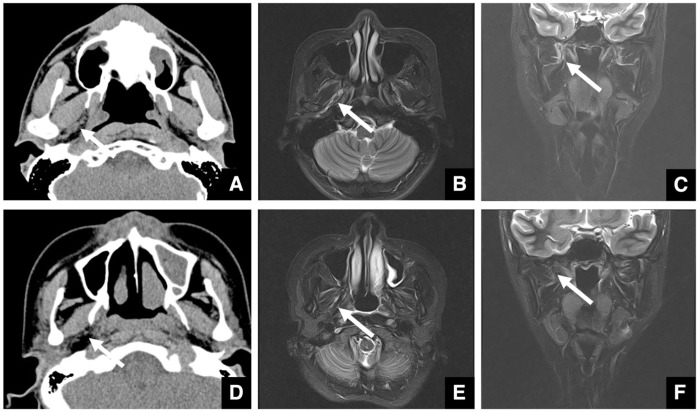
MDCT (slice thickness, 3.0 mm) and MR images of the pterygoid venous plexus. (A) Axial soft tissue MDCT image of a well-developed pterygoid venous plexus. Vascular structures (arrow) are prominent within fat tissue. Mucosal cysts are seen incidentally in the left maxillary sinus. (B) Axial MRI short tau inversion recovery (STIR) image (TR 4700 ms; TE 75 ms; slice thickness 6 mm) of the same patient as in (A). High signal intensity is observed within the fat tissue (arrow). (C) Coronal MRI STIR image of the same patient as in (A). High signal intensity is observed within the fat tissue (arrow). (D) Axial soft tissue MDCT image of an underdeveloped pterygoid venous plexus. Vascular structures (arrow) appear sparse within the fat tissue. Mucosal cysts are seen incidentally in the left maxillary sinus. (E) Axial MRI STIR image of the same patient as in (D). No high signal intensity is observed within the fat tissue (arrow). (F) Coronal MRI STIR image of the same patient as in (D). No high signal intensity is observed within the fat tissue (arrow). Permission has been obtained to publish all figures.
